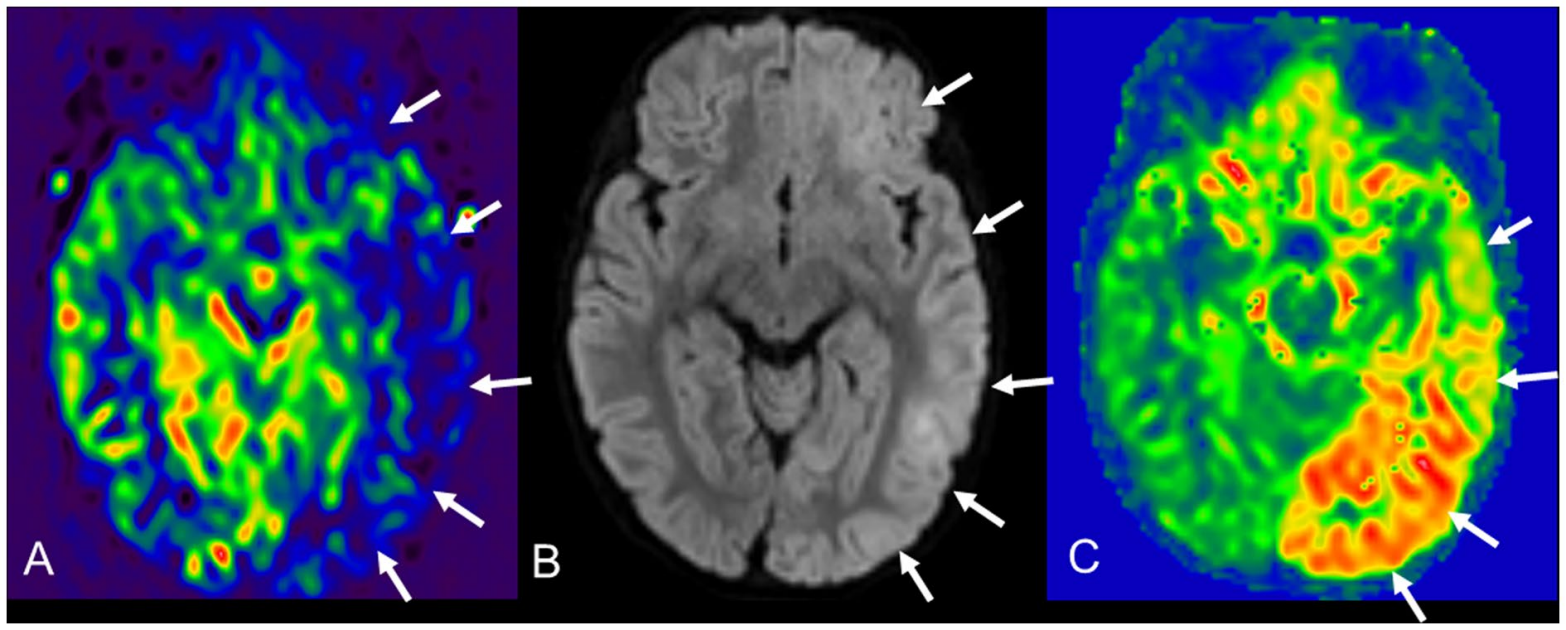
A 22-month-old AESD male with residual psychomotor retardation. On day 1, DWI and other conventional sequences show normal findings, but pulsed ASL (A) shows moderate hypoperfusion in the left temporal, occipital, and frontal cortex asymmetry (arrows). He was treated with targeted temperature management with ventilator and sustained sedation. Late seizures did not occur owing to treatment, but DWI (B) and pulsed ASL (C) 5 days after early seizures show apparent BTA and moderate hyperperfusion (arrows) in the left subcortical region, where ASL exhibited hypoperfusion on day 1. AESD, acute encephalopathy with biphasic seizures and late reduced diffusion; DWI, diffusion-weighted imaging; ASL, arterial spin labeling; BTA, bright tree appearance.
